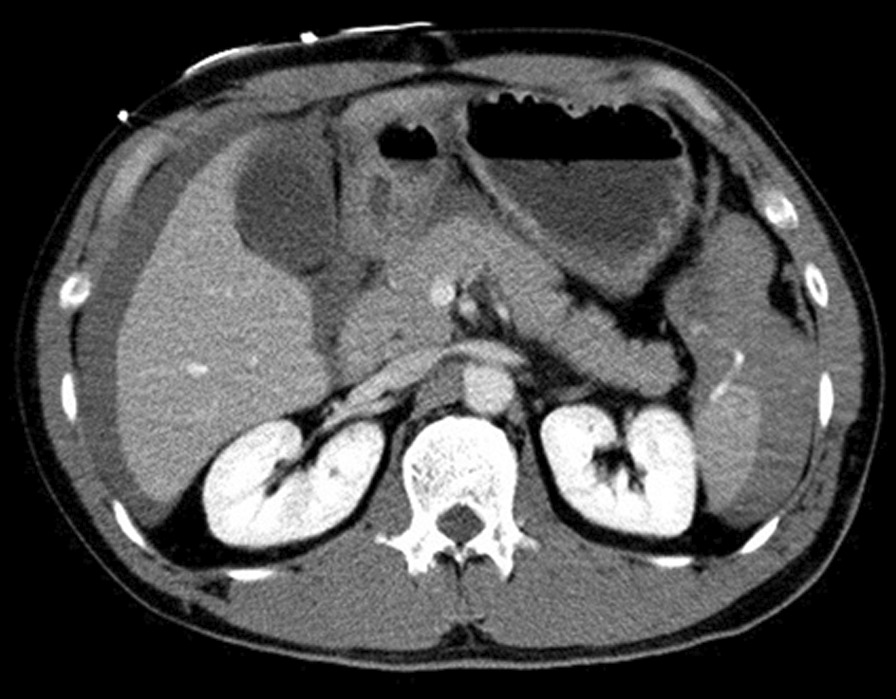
Abdominal computed tomography indicated traumatic splenic injury and intra-abdominal hemorrhage. Contrast extravasation from the lower pole of the spleen was observed
